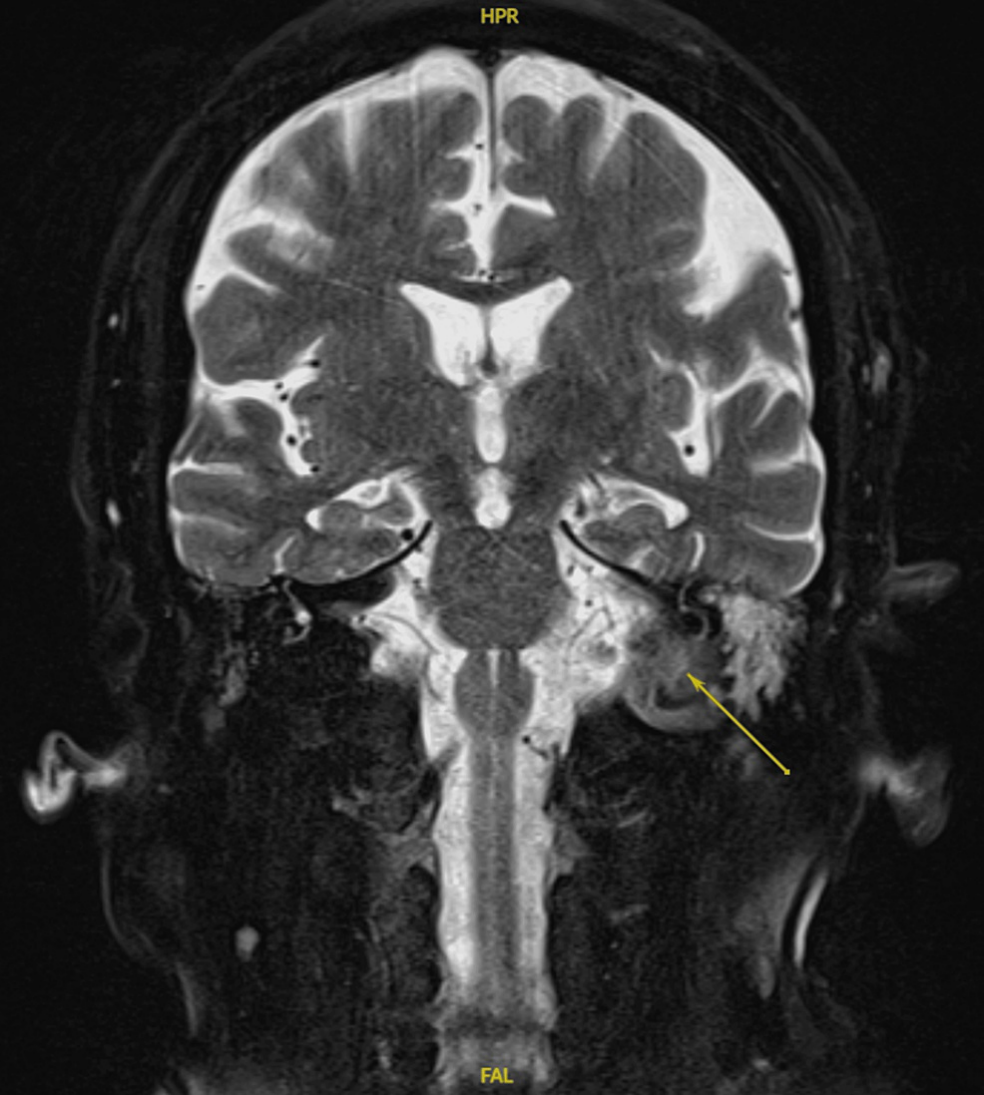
Coronal MRI IAM showing marked soft tissue thickening, oedema in the left EAC, retrocondylar space, parapharyngeal space and carotid space (indicated by an arrow). IAM: Internal auditory meatus; EAC: external auditory canal
